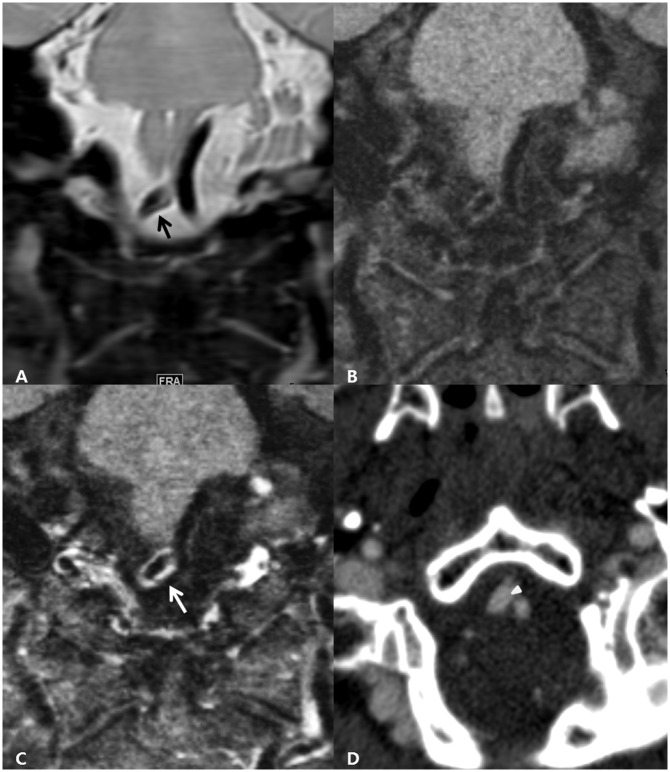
A 42-year-old man presenting with a headache. The coronal image of 3D-PD (A) clearly demonstrates the intimal flap (black arrow) which is not identified on the pre- (B) and post-contrast (C) 3D-iMSDE-T1 images. Abnormal vascular enhancement (white arrow) is revealed on the coronal post-contrast 3D-iMSDE-T1 image. The CTA source image (D) shows the intimal flap (white arrowhead).
